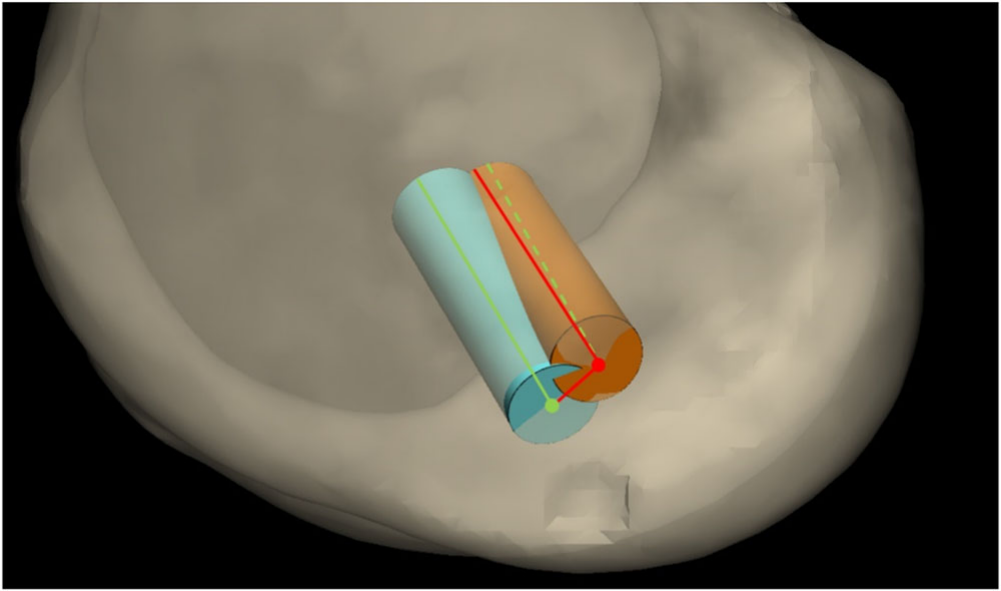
Overlay of 3D reconstructions made from pre‐ and post‐operative CT scans. Measurements of the entry point distance and angular deviation between the planned (blue) and post‐operative (orange) tunnels. CT, computerized tomography.
